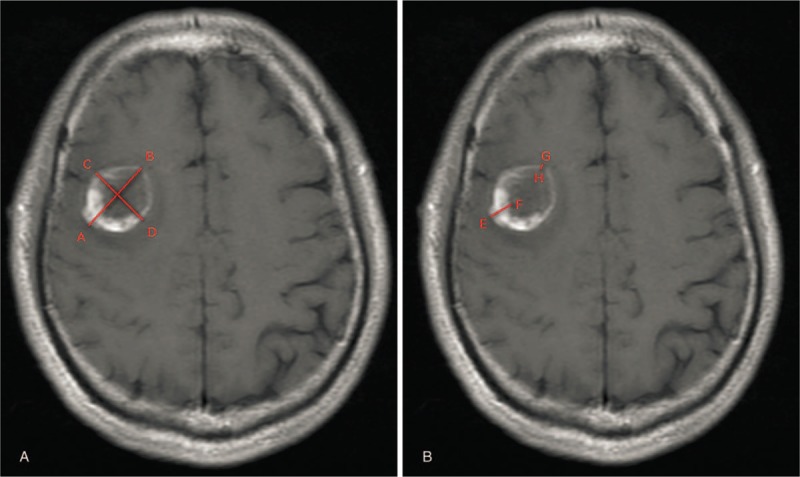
Example of an image for diameter and thickness measurement in cMRI (enhanced T1WI). (A) Line AB and line CD represent LDE and SDE, respectively. The LDN and SDN are measured in the same way as LDE and SDE but in the necrosis portion. (B) Line EF and Line GH represent the MaxiTE and MinTE, respectively. cMRI = conventional magnetic resonance imaging, LDE = long diameter of the enhanced portion, LDN = long diameter of the necrotic portion, MaxTE = maximum thickness of the enhanced portion, MinTE = minimum thickness of the enhanced portion, SDE = short diameter of the enhanced portion, SDN = short diameter of the necrotic portion.
